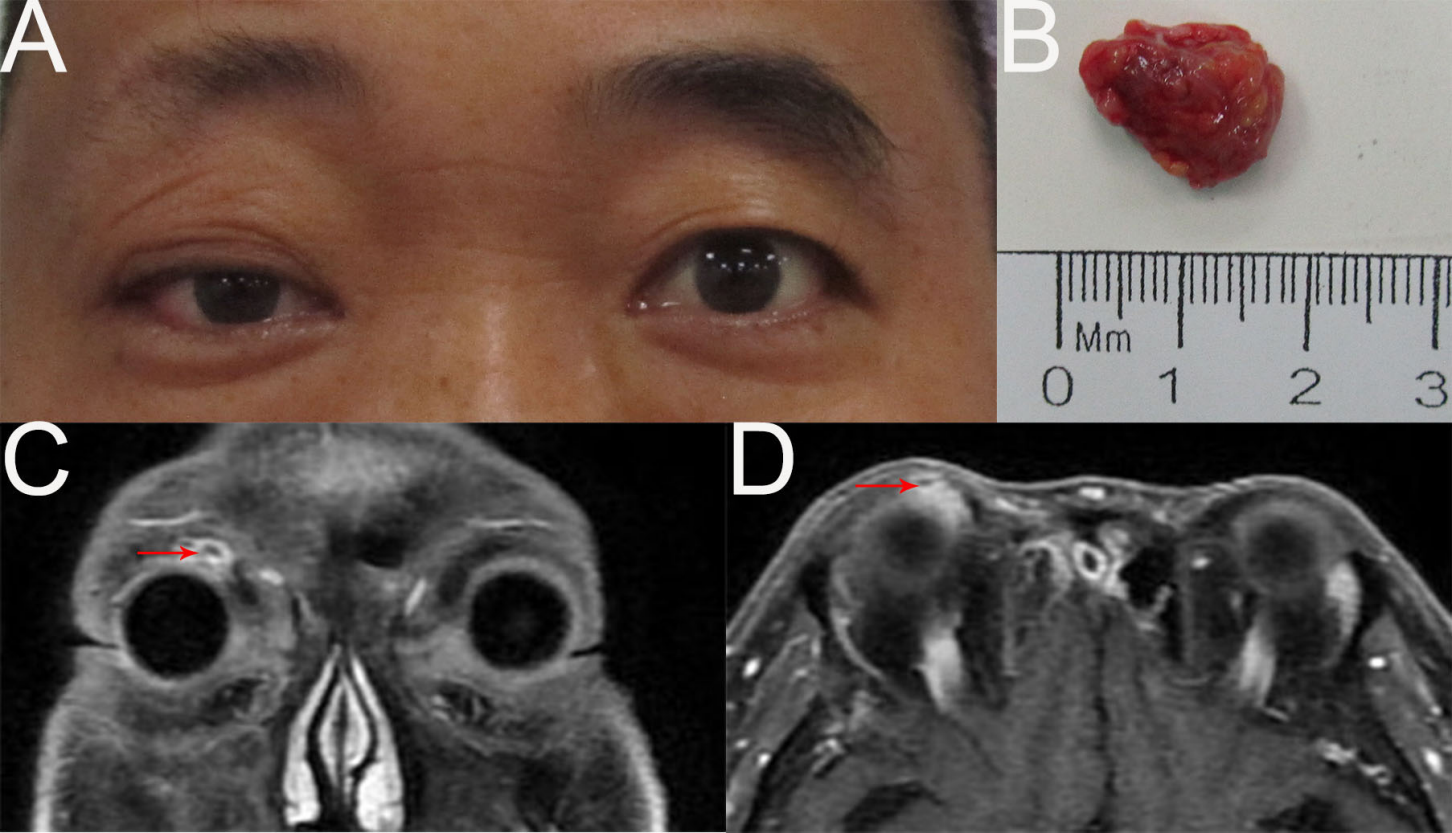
(A) External image of the first recurrence. (B) The mass was dissected under the table and was approximately 1.7cmx1.2cmx0.8cm in size. (C, D) Contract-enhanced MRI with STIR sequence of the orbit showed the absence of the right lacrimal gland and an oval abnormal signal (1.0cm×0.9cm) in the right superomedial orbit with peripheral rim enhancement.
